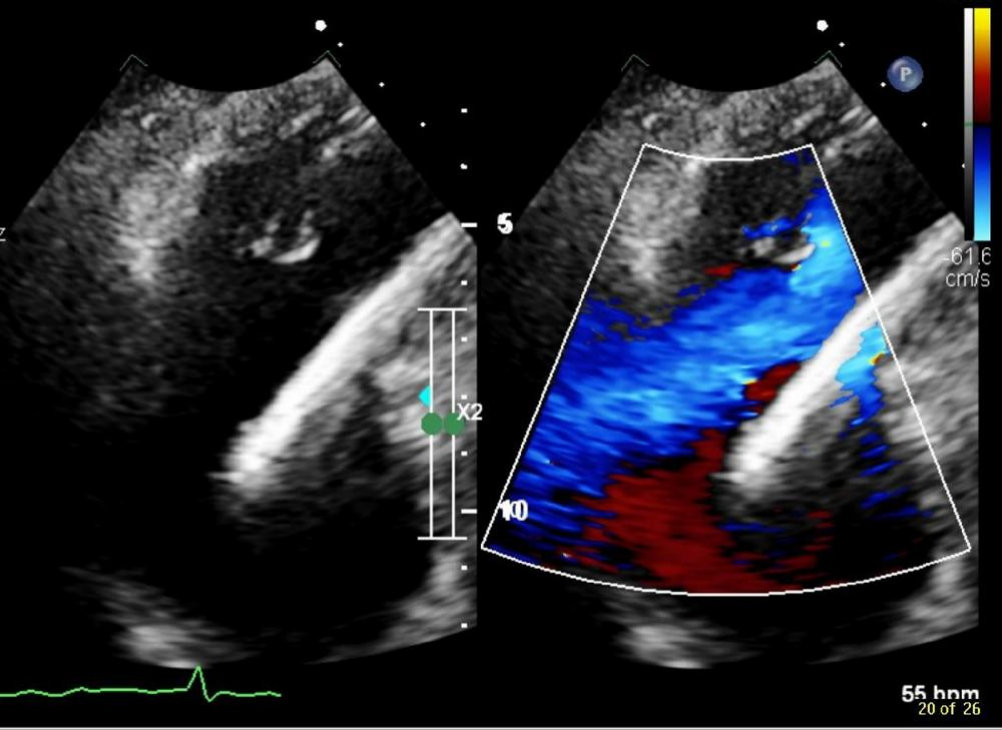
Apical four-chamber view, zoom on the tricuspid valve. Right ventricular pacing lead splinting open the septal leaflet causing a large coaptation gap.
